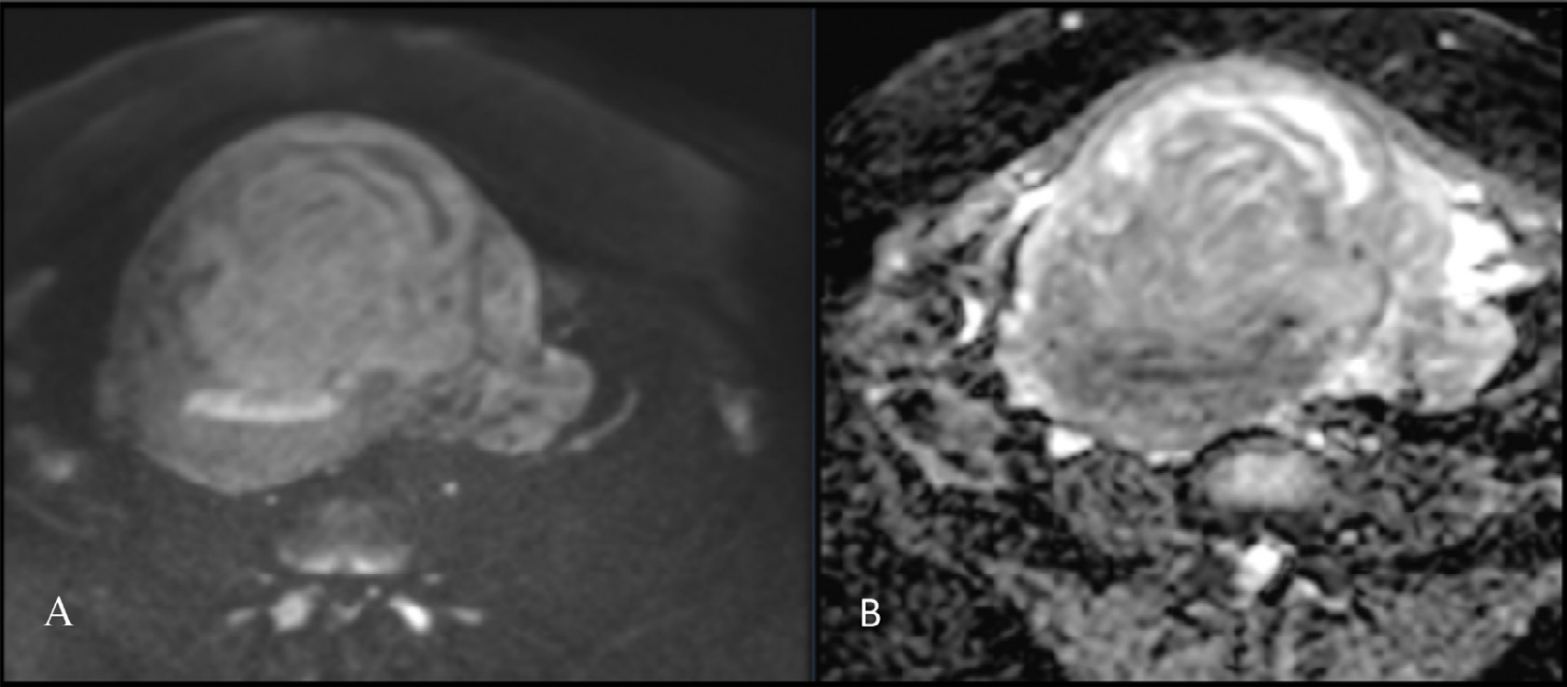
Axial DWI (*b* = 1000 s/mm²) (A) and corresponding ADC map (B) demonstrating the myometrial mass with high signal intensity on DWI and correspondingly high ADC values, indicating no diffusion restriction.
